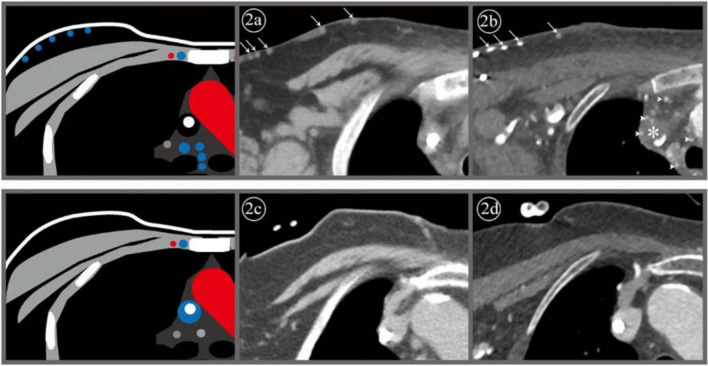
Positive LTP: Vascular-like attenuation was observed in the anterolateral chest wall on low-dose CT (a, arrows). The vascular-like attenuation shown by low-dose CT was confirmed in the CT venography (b, arrows). Note the protein sheath around the central venous catheter resulting in stenosis of the superior vena cava (asterisks), along with multiple collateral veins developing in the mediastinum (arrowheads). Negative LTP: No vascular-like attenuation of the anterolateral chest wall was present on low-dose CT and CT venography (c,d).
